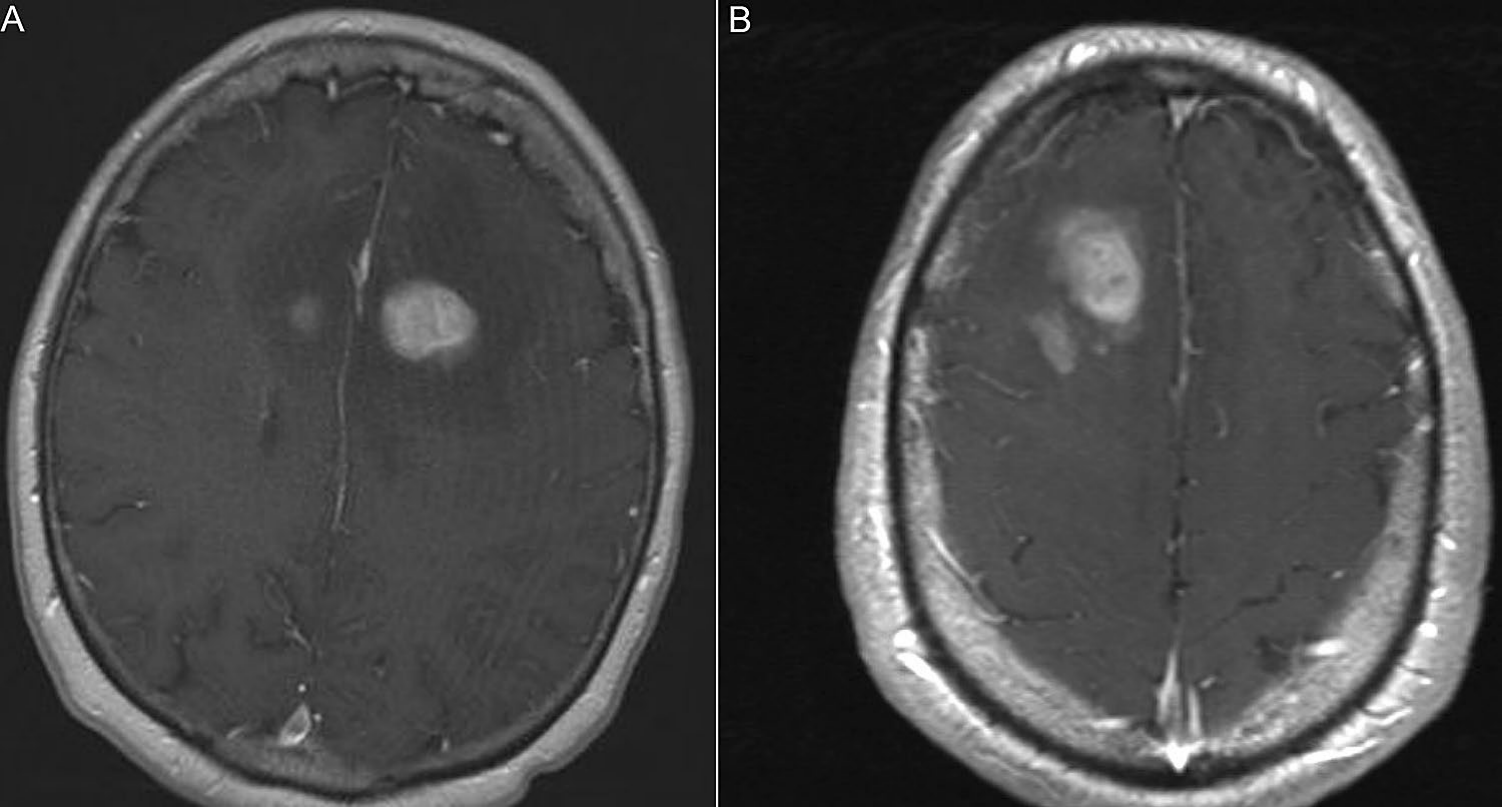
Representative MR images for classification. (A) Contrast-enhanced T_1_-weighted image of a 71-year-old woman with primary central nervous system lymphoma in the right thalamus. (B) Contrast-enhanced T_1_-weighted image of a 70-year-old man with primary central nervous system lymphoma in the right frontal lobe.
